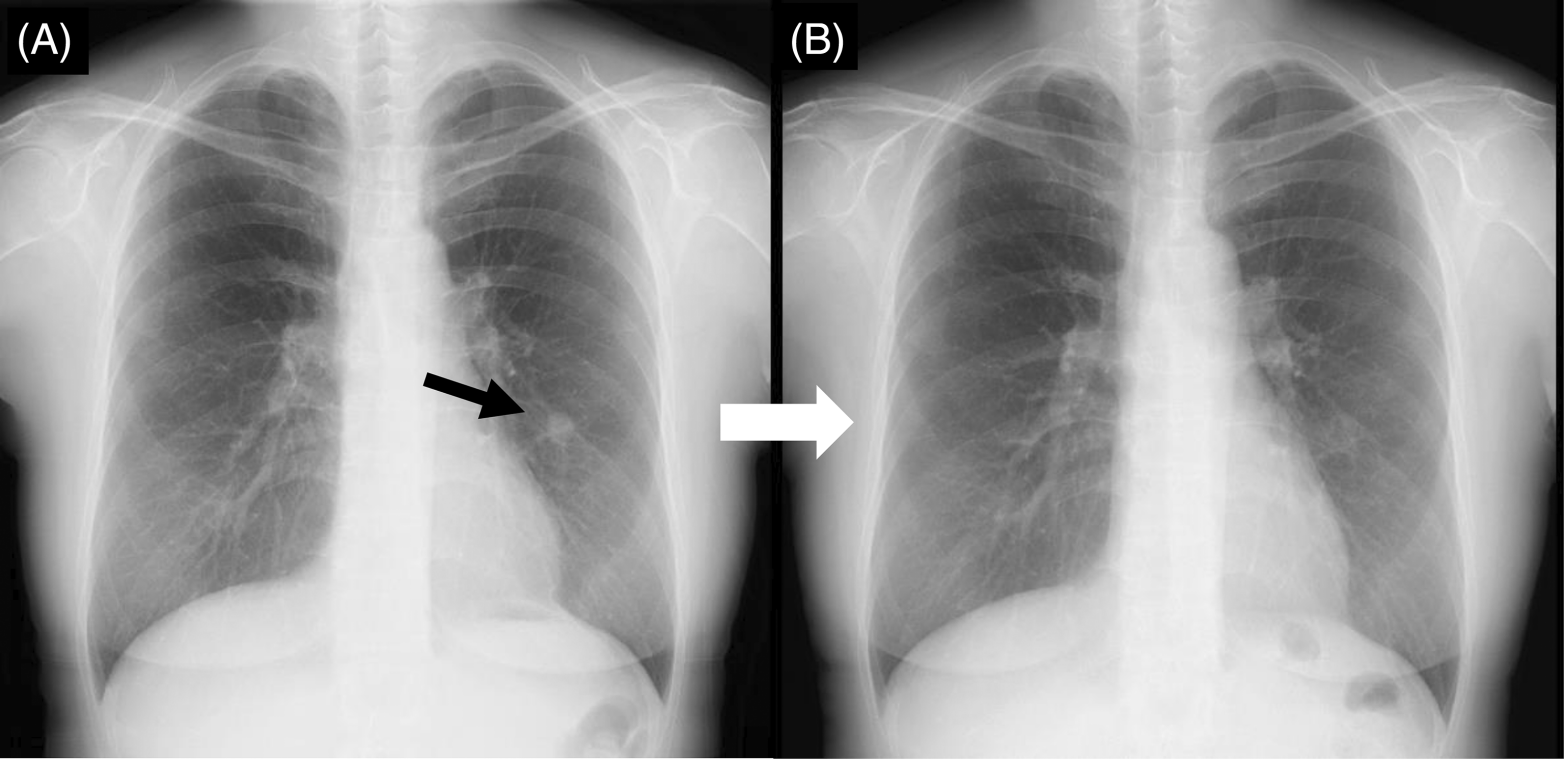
Radiographic imaging showed the disappearance of the nodule (black arrow) 3 months after bronchoscopic removal.(A: Chest x‐ray on admission and B: 3 month after bronchoscopic removal).
